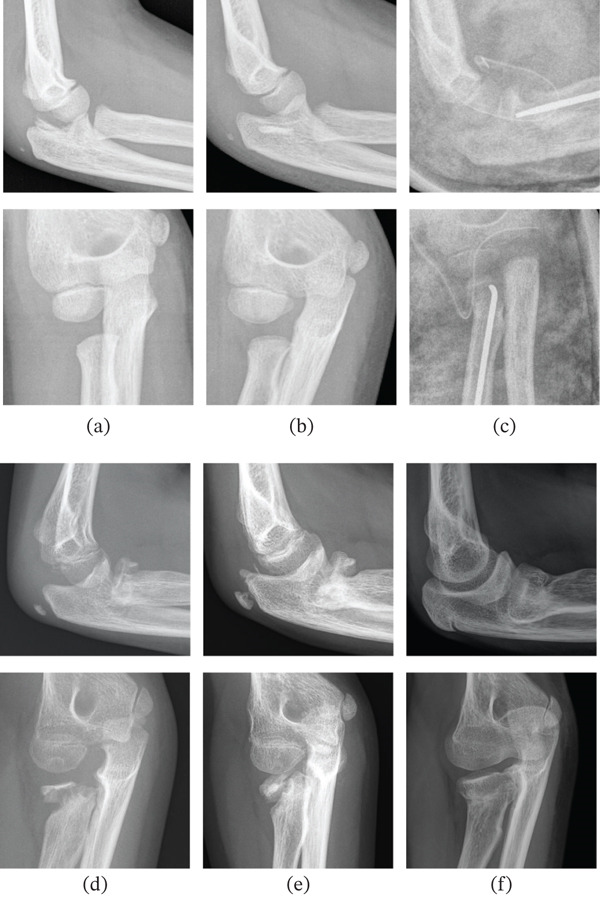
Radiographic evolution of Case 2 (lateral views in the upper row and anteroposterior views in the lower row): (a) acute Jeffery lesion of the right elbow, (b) x‐ray 3 weeks postinjury, (c) open reduction and osteosynthesis with elastic nail, (d) removal of osteosynthesis material at 8 months, (e) Follow‐up at 2 years and (f) follow‐up at 3 years.
